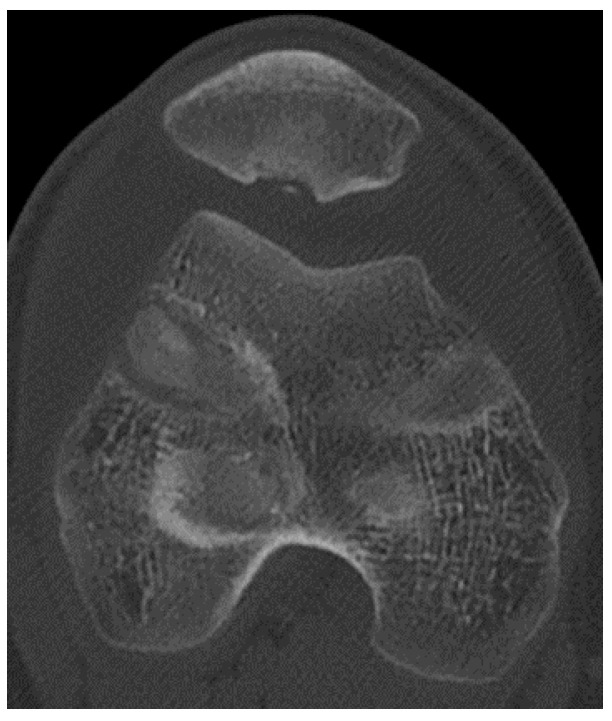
CT scan image of an osteochondral lesion of the patella.
